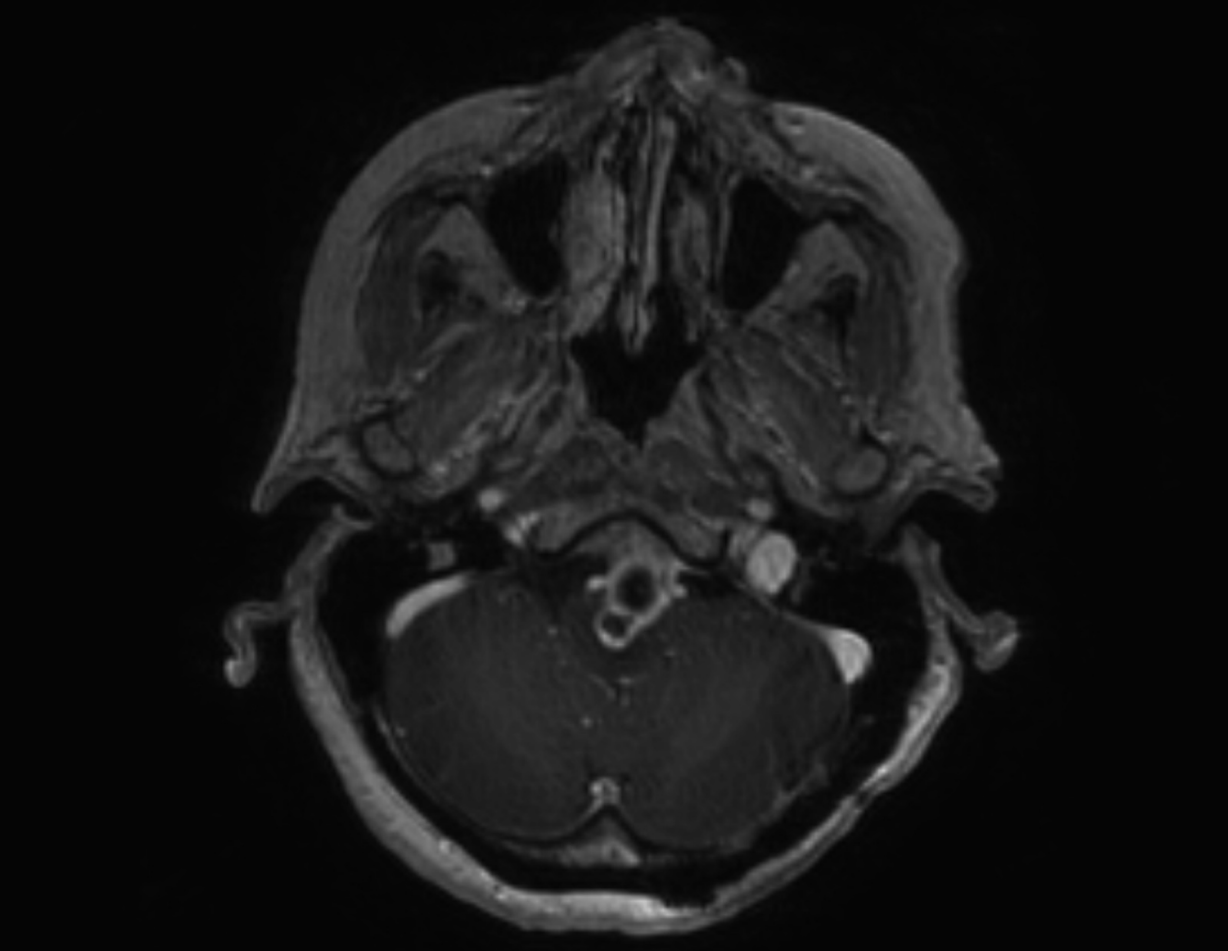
September Axial MRI Image 1 Posterior fossa
